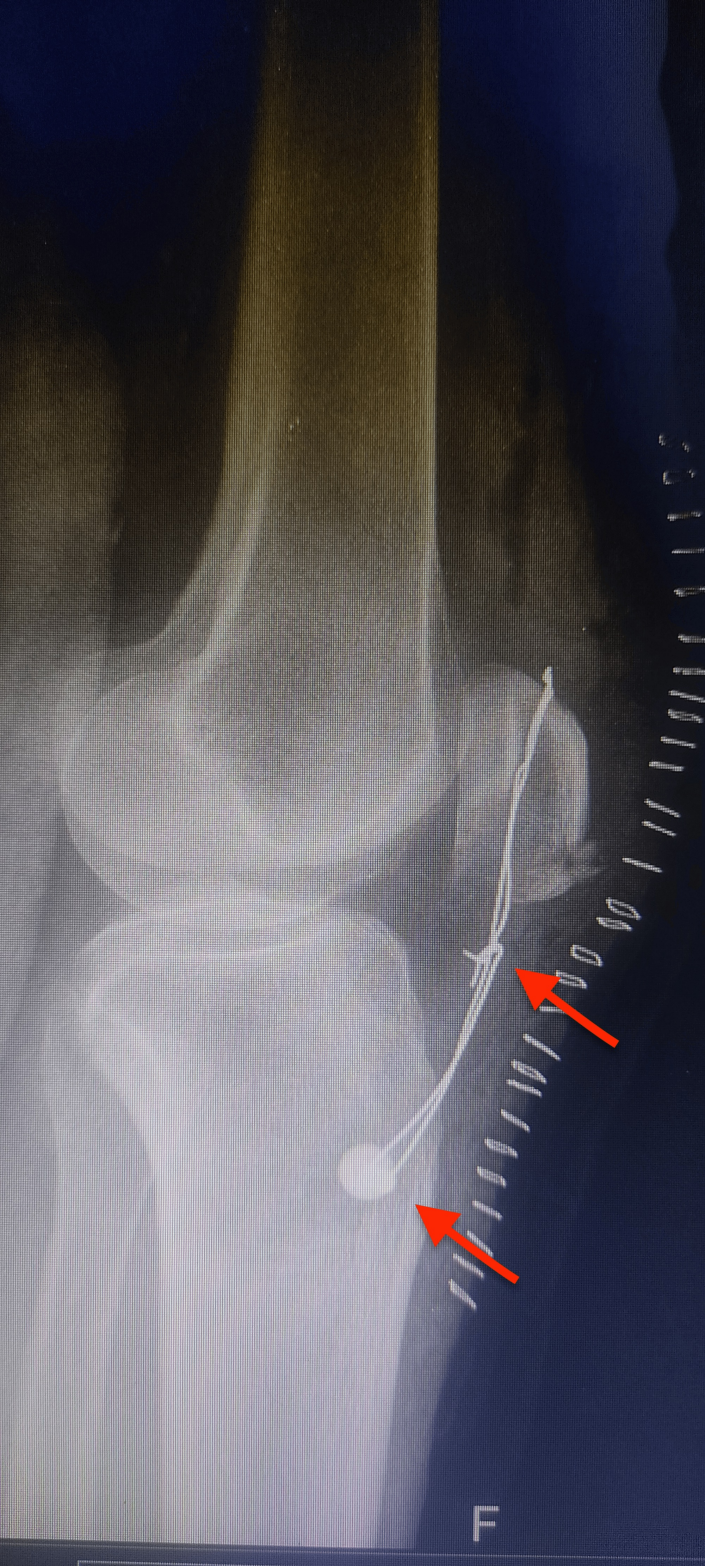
Lateral X-ray of the left knee after partial patellectomy with screw fixation to tibia and tension band construct.
